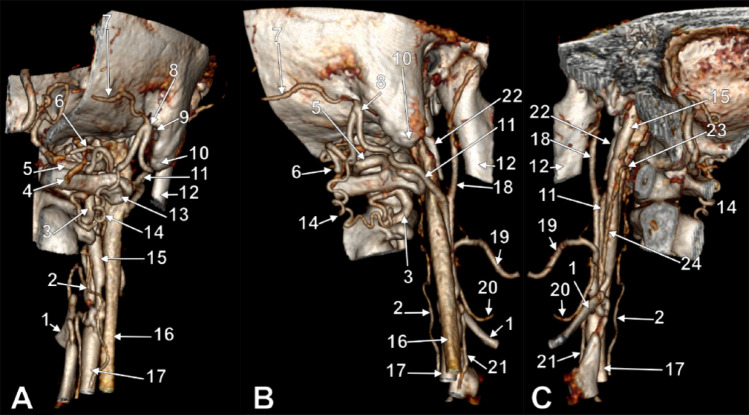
CTA 3D volume-rendered reconstruction of the right cervical and suboccipital regions showing the persisting carotid duct (PCD) and the persisting proatlantal artery (PPA). A: postero-infero-medial view; B: postero-infero-lateral view; C: medial view. (1) greater hyoid horn; (2) ascending PCD; (3) V2 vertebral artery (VA); (4) posterior condylar vein; (5) V3 VA; (6) anterior br.of PPA ; (7) occipital artery; (8) superior loop of PPA; (9) mastoid notch; (10) mastoid tip; 11. PPA; 12. mandibular ramus; 13. transverse process C1; 14. inferior br. of PPA; 15. internal carotid artery; 16. internal jugular vein; 17. common carotid artery; 18. external carotid artery; 19. facial artery; 20. lingual artery; 21. superior thyroid artery; 22. styloid process; 23. preatlantal loop of PCD; 24. descending PCD
